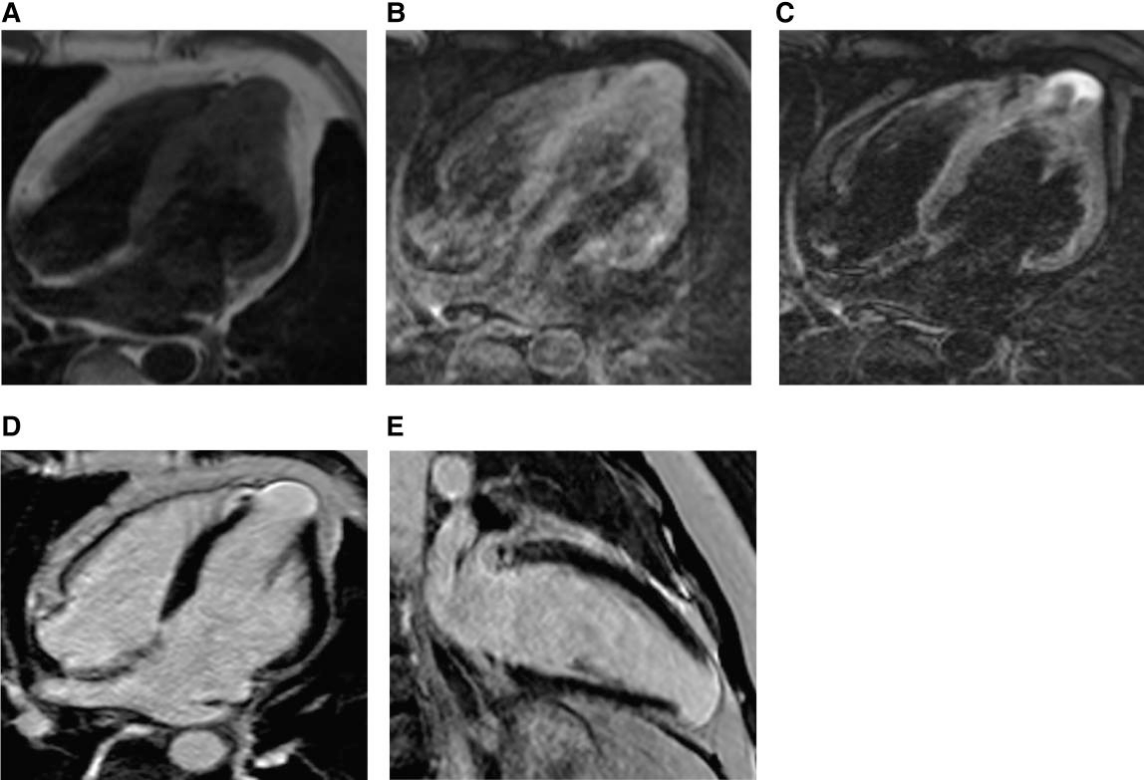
Cardiac magnetic resonance imaging (2020.09) revealed intramyocardial fatty spots in the left ventricle, especially at the apex. (*A* and *B*) T1-weighted black blood imaging with and without fat suppression. T1WI showed increased fat signal at the LV apex; (*C*) T2WI revealed a significant increase in apical signal with fat suppression. (*D* and *E*) Late gadolinium enhancement in LV apex.
